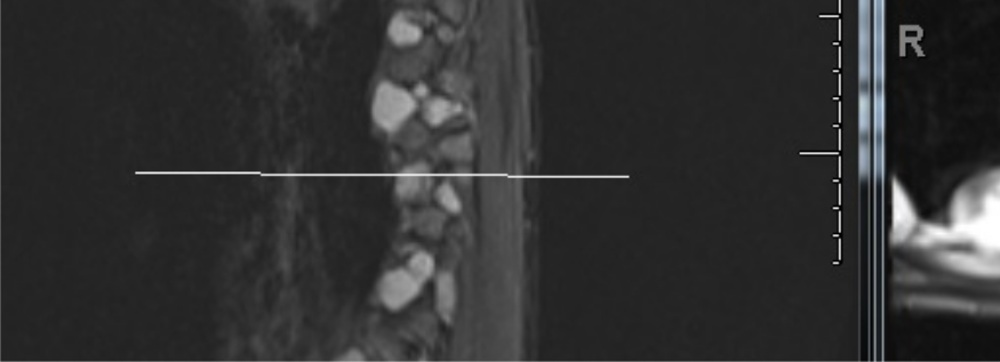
MRI of the thoracic spine with multilevel right-sided foraminal lesions.
